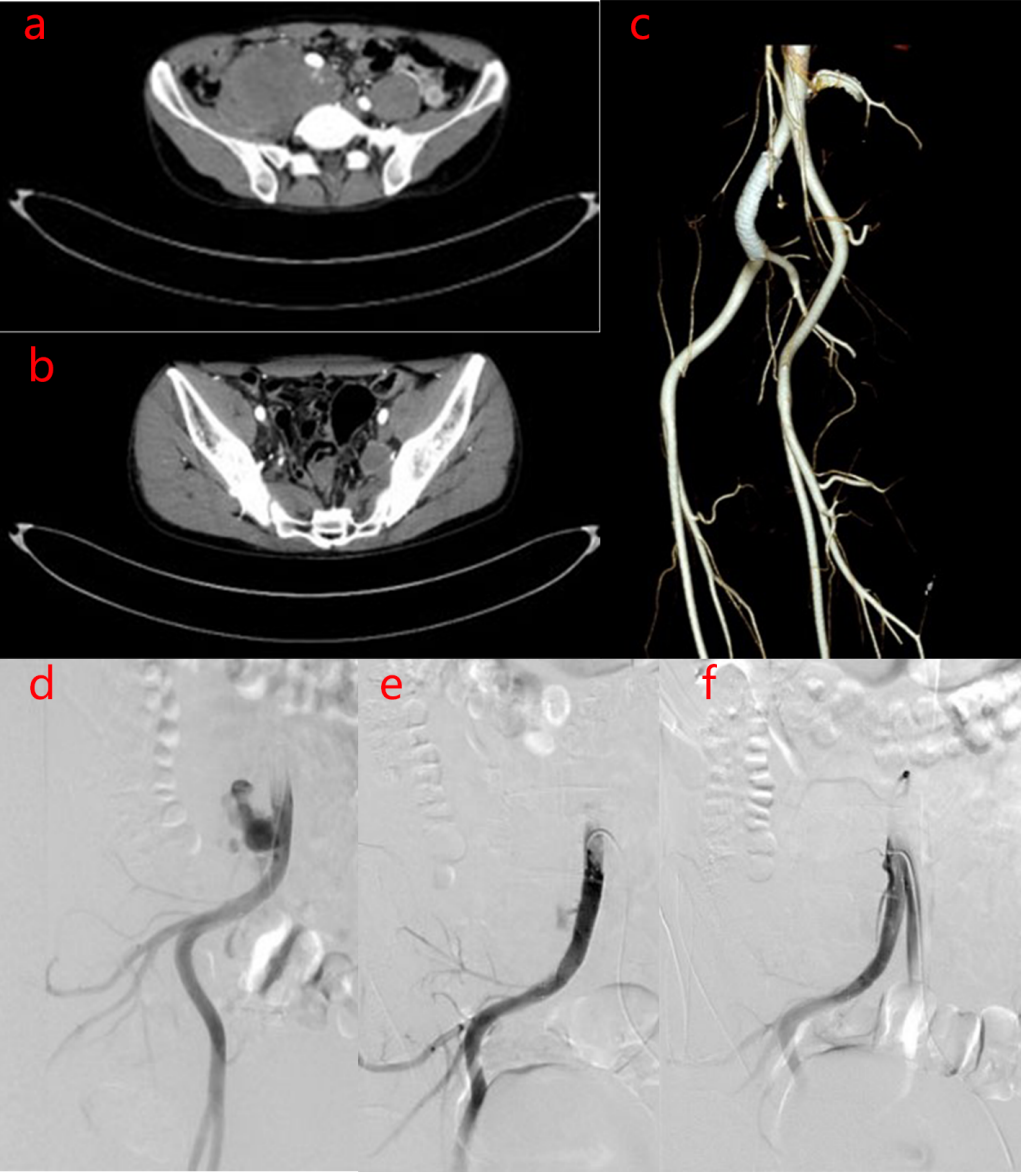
Imaging manifestations of pseudoaneurysm of the right CIA before and after covered stent implantation. (A–C). After 27 months of bare stent implantation, abdominal CTA showed the following findings: The size of the pseudoaneurysm was obviously increased compared to that post-operation, because the contrast agent continuously flows into the cavity of the pseudoaneurysm. However, there was no contrast agent in the left IIA pseudoaneurysm area. (D–F). After covered stent implantation, DSA of the right inferior vena cava showed the following findings: There was less contrast agent blowing into the cavity of the pseudoaneurysm of the right CIA and the size of the pseudoaneurysm was decreased.
